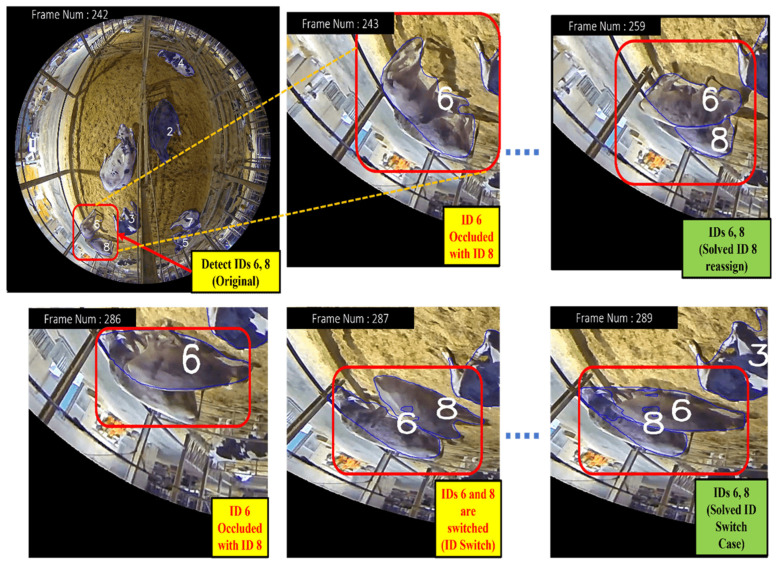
Sample performance analysis of miss detection and occlusion using CTA.
